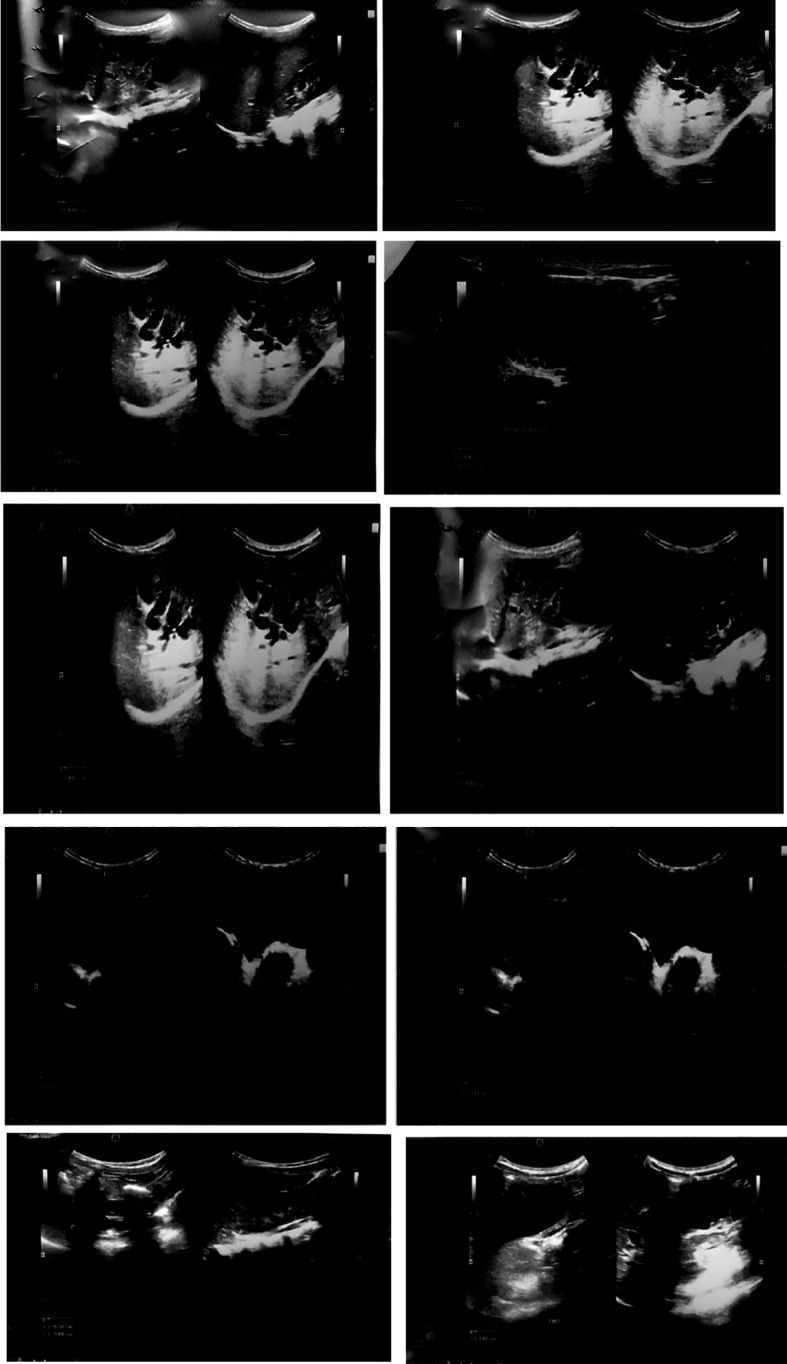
Multiple dilatation of both intrahepatic and extra hepatic biliary duct in keeping with the Type IV A cysts at ultrasonography.
